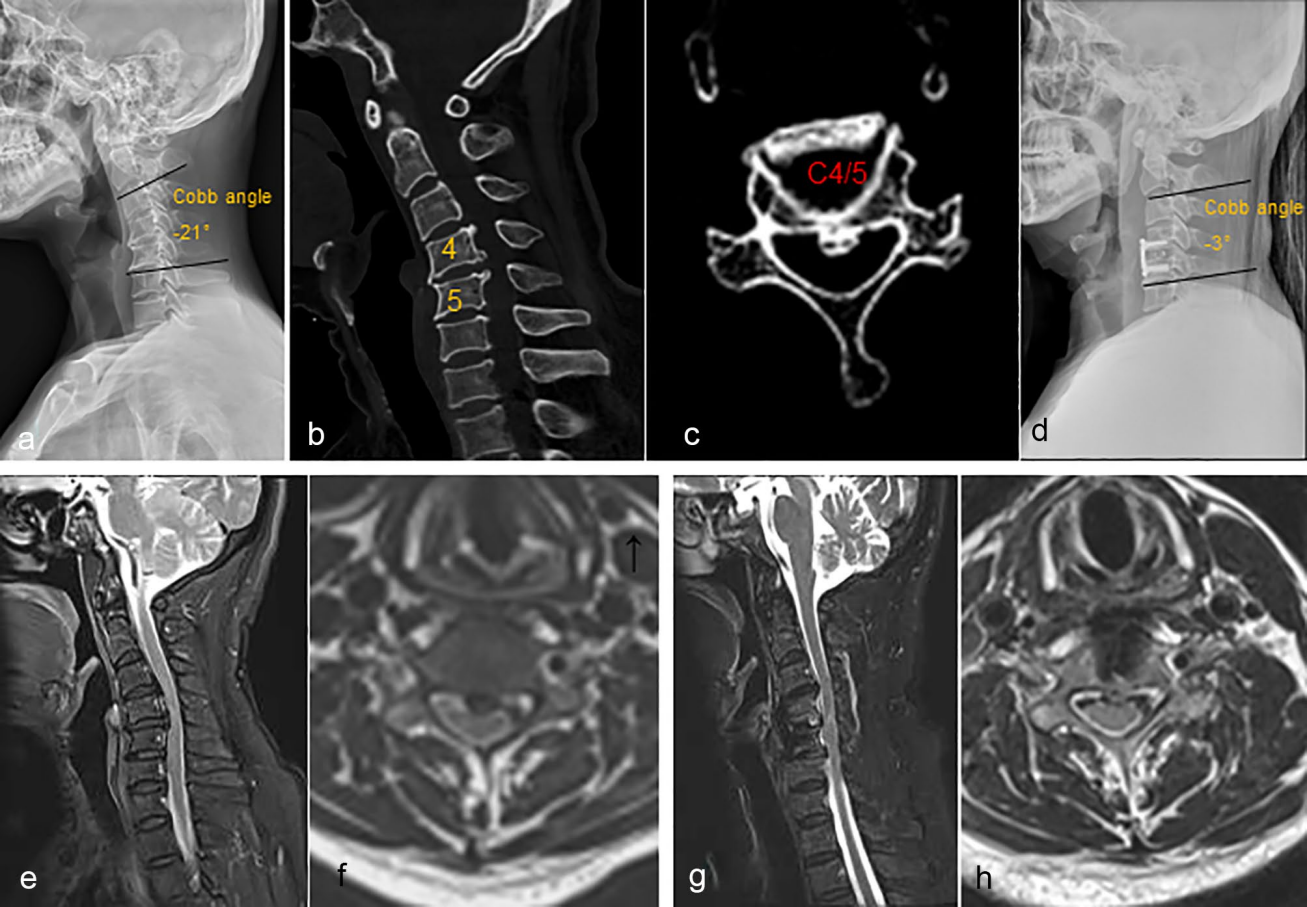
Case 25: a patients was 58 years, male, the walking unstable was main clinical symptom, the compression spinal cord due to herniation of intervertebral disc and cervical kyphosis, X-ray (a), CT (b,c) and MRI (e,f) before the ACDF. We performed single-level ACDF at C4/5, that decompressed spinal cord and corrected the cervical kyphosis (d,g,h). The walking unstable gradually improved during postoperative follow-up.
